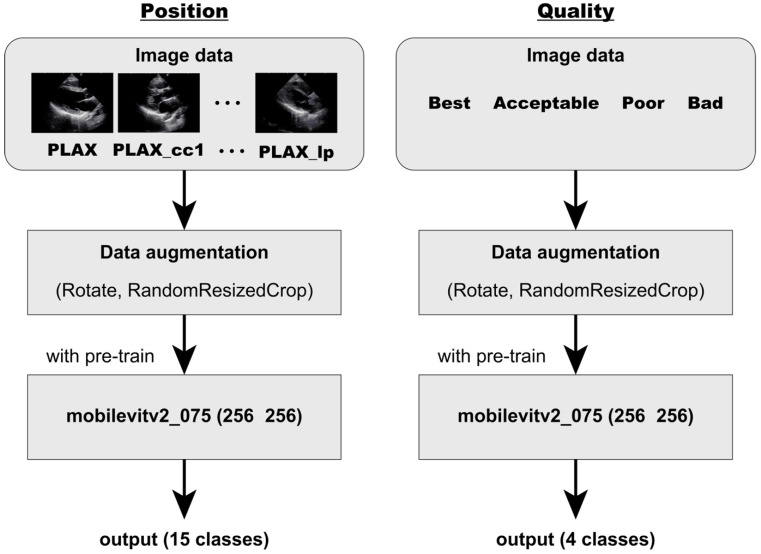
Training Methods for Position and Quality Evaluation Models. The figure shows the training process for the position evaluation model (parasternal long-axis view) and the quality evaluation model. Training images were subjected to data augmentation with a 30% probability and then input into the mobileViTv2_075 model. The model outputs the class probabilities for each evaluation.
